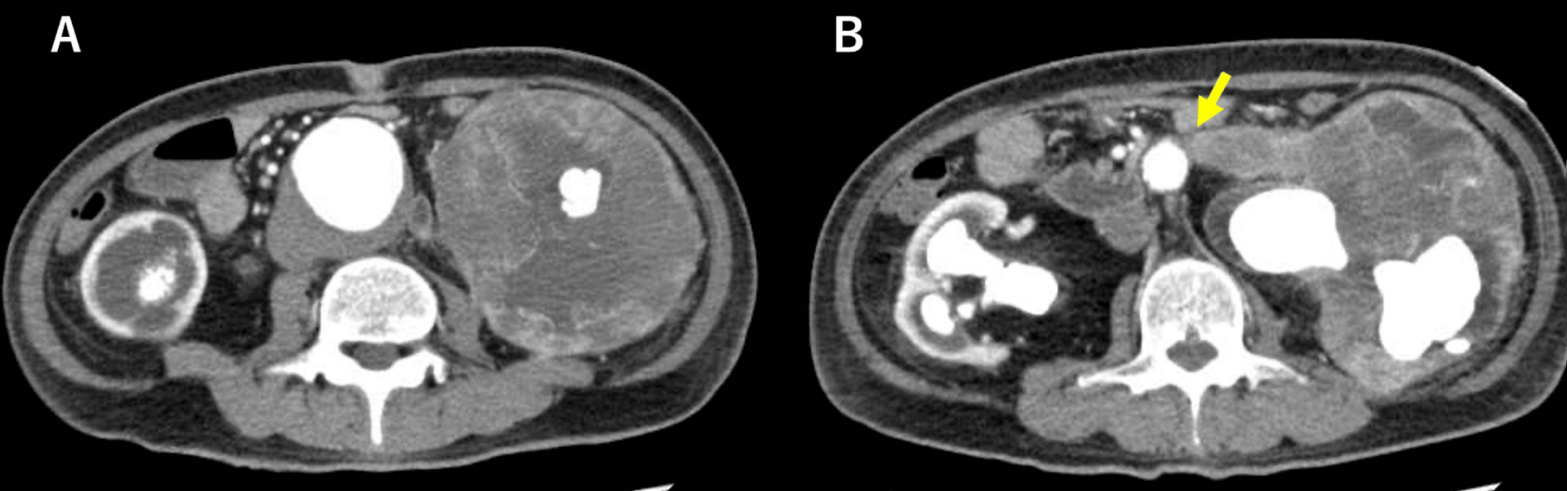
Contrast-enhanced CT A: Giant left RT and infrarenal AAA 58 mm in diameter. B: The RT is adjacent to the aortic wall (arrow) AAA: abdominal aortic aneurysm; CT: computed tomography; RT: renal tumor
